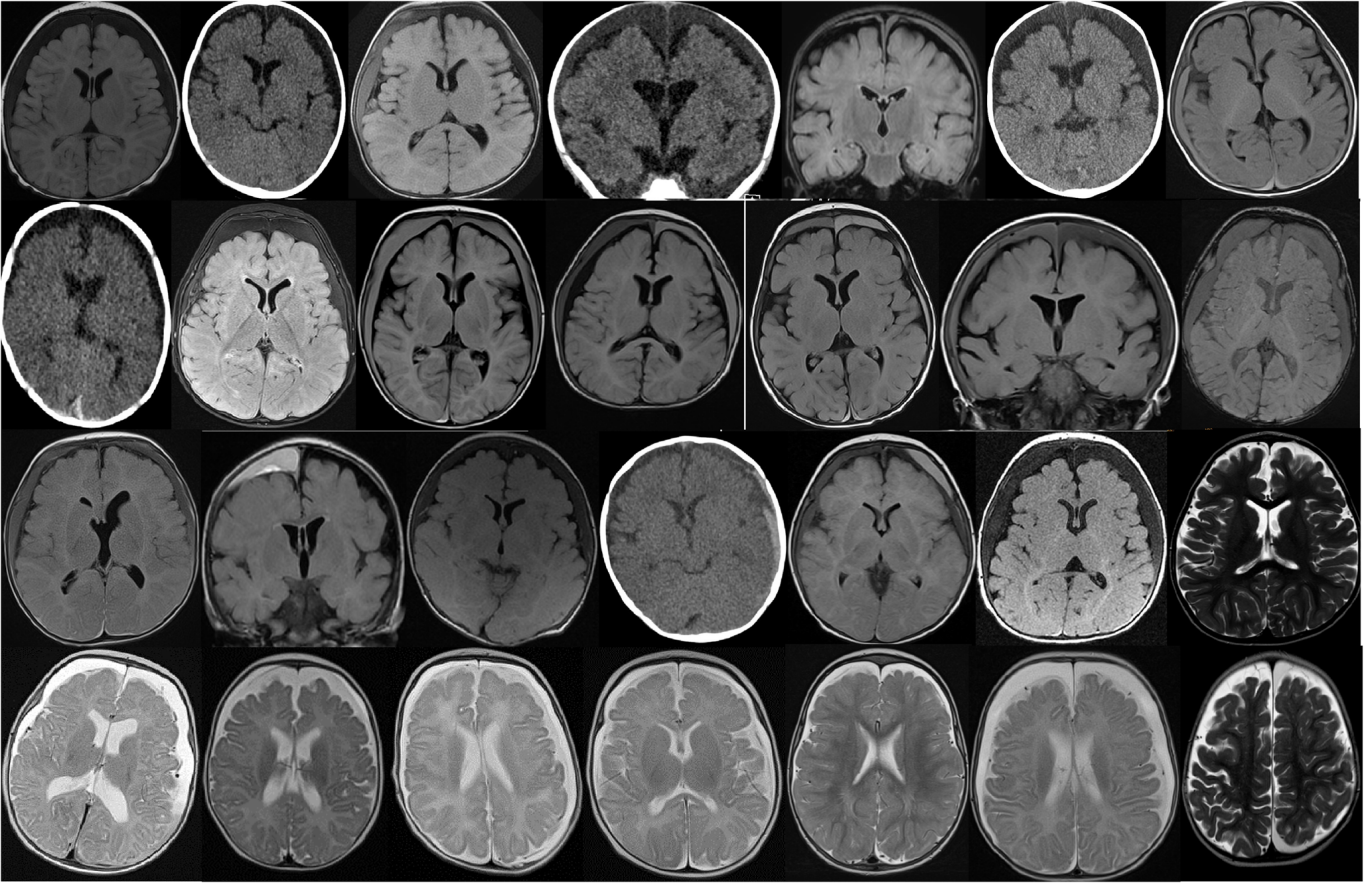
CT or MRI images approximately at the level of the foramina of Monro for 27 of the infants and one image closer to vertex for pat #28 (lower right). The patients are displayed in the following order, from left to right: upper row pats. # 1–7, second row: pats. #8–14, third row: pats. #15–21, and bottom row: pats. #22–28
